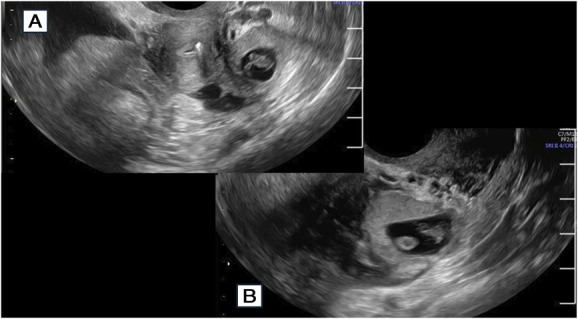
Ultrasonogram images of left adnexal ectopic pregnancy with adjacent uterus. Transverse image (A) and longitudinal image (B).
